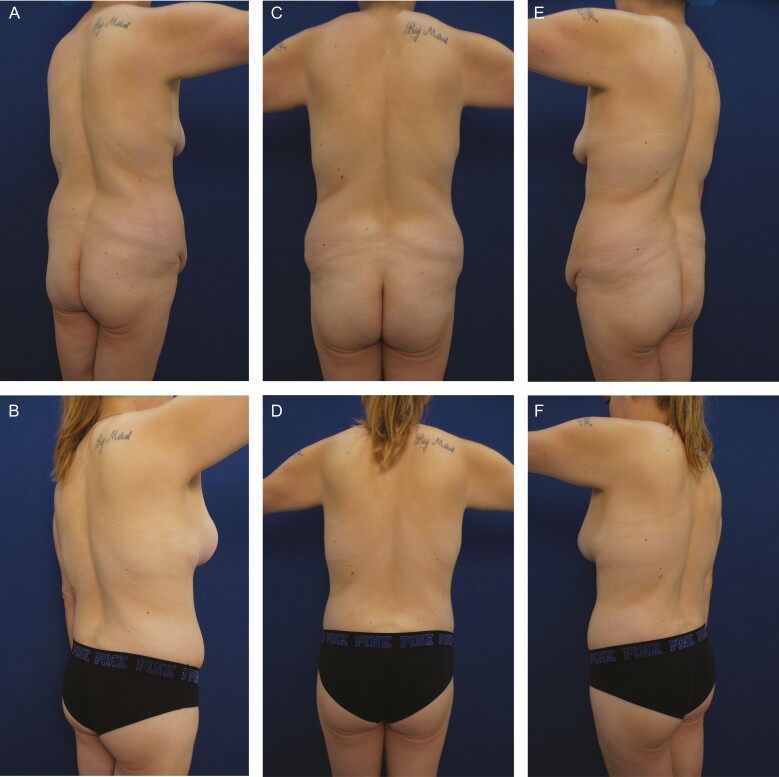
Elimination of back rolls following ultrasound-assisted liposuction and helium-activated radiofrequency treatment. This 35-year-old female patient demonstrates skin redundancy and excess fat, which led to the formation of a mid-back roll. Successful treatment required liposuction with infiltration and aspiration of 100 cc tumescent solution per side as well as skin tightening using subdermal coagulation. Photographs were taken before and 3 months after surgery. Preoperative and 3 months postoperative (A, B) right lateral view, (C, D) posterior view, and (E, F) left lateral view.
